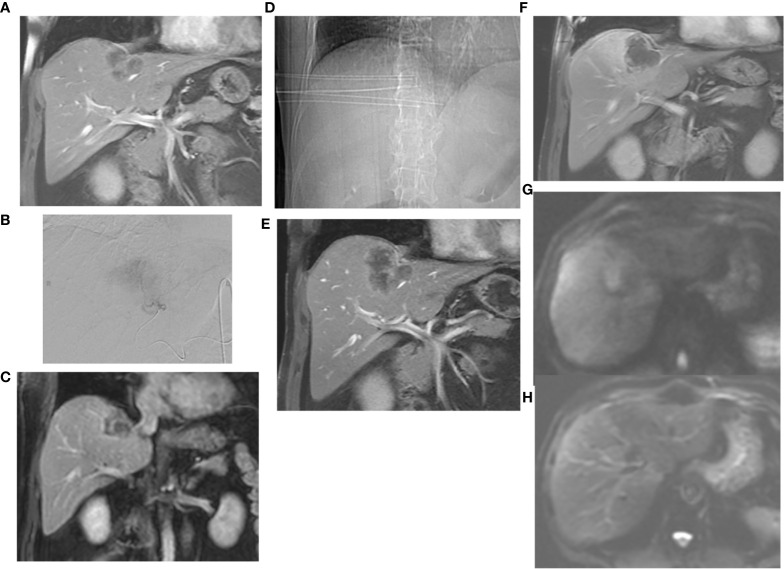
(A) Under third-line systemic chemotherapy, progressive solitary, surgically unresectable colorectal liver metastasis on the border between segment IVa and VIII. (B) The lesion has been initially transarterially chemoembolized with DEB-IRI (Irinotecan-loaded drug-eluting beads). (C) contrast-enhanced MRI shows subtotal devascularisation of the metastasis with a still vital tumour margin on the right lateral-apical side. (D) The marginal recurrences have been interstitially brachytherapied in the interval. The topogram clearly shows the parallel positioning of the applicators. (E) Excellent local tumor control was observed after 3 months. (F) timely shrinkage of the metastasis as well as further local tumor control without detection of recurrence after 6 months. (G) Local tumor control also confirmed after 12 months by the absence of diffusion restriction in DWI. (H) The corresponding ADC-maps.
